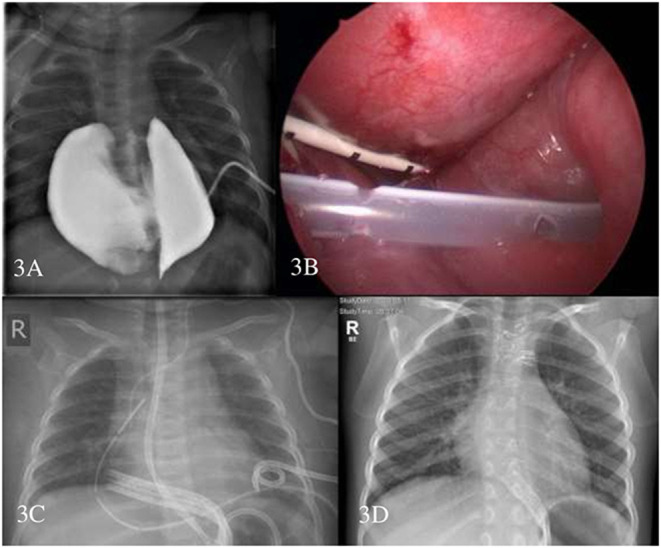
Patient #3. Contrast filling of the intrathoracic central conducting lymphatic anomaly (CCLA) through the pigtail catheter to depict the anatomic relationship (A) thoracoscopic placement of the Denver drain—pigtail drain is seen (B) chest X-ray with multiple drains (C) and chest X-ray 1-year after sternotomy and surgical resection of CCLA (D).
